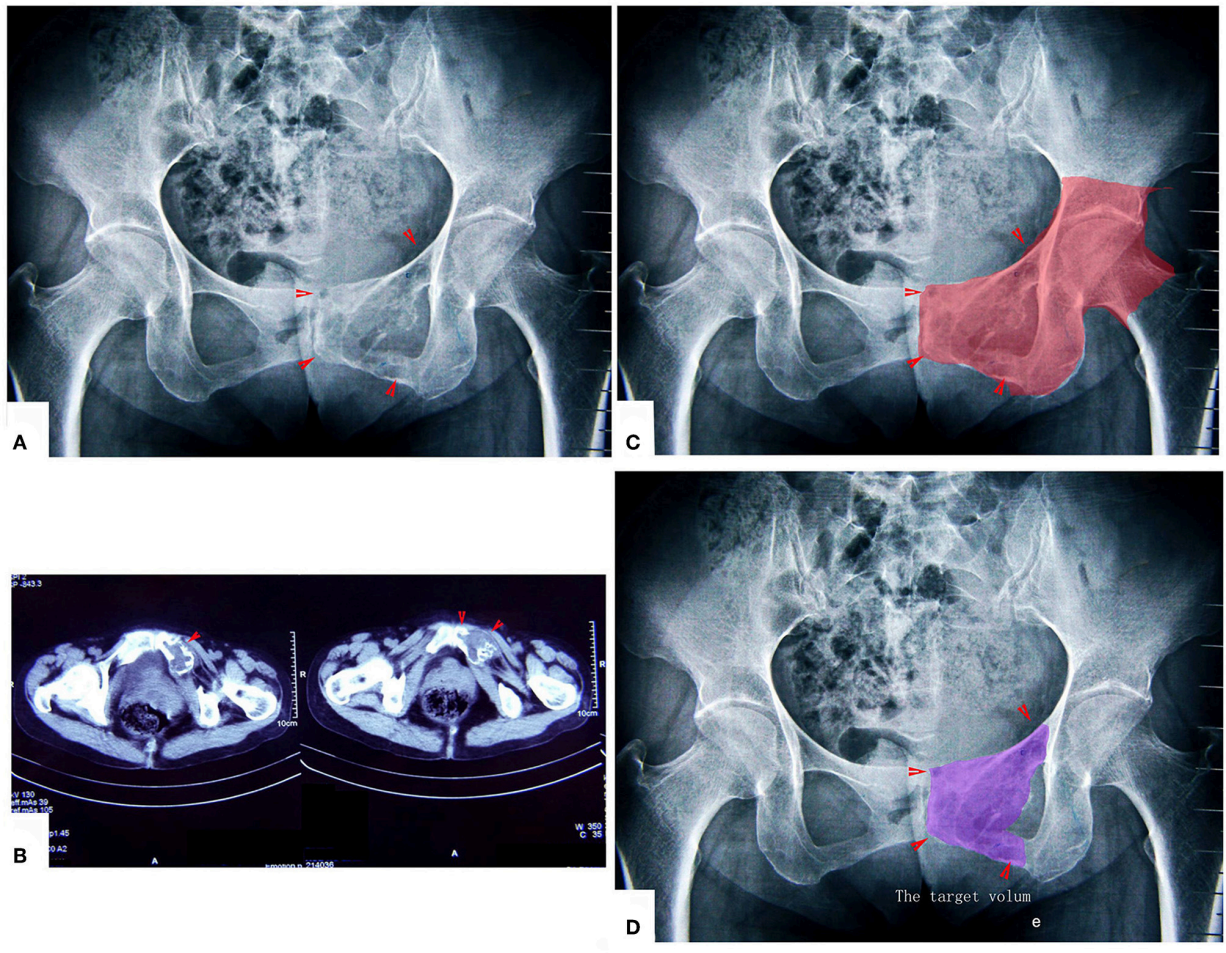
A patient with chondrosarcoma of the pecten pubis and inferior pubic ramus. (A,B) X-ray and CT scan. The lesion was near the inner wall of the hip joint. (C) The colored region shows the range of traditional treating method (en bloc resection). (D) The colored region shows the target volume for MWA treatment.
